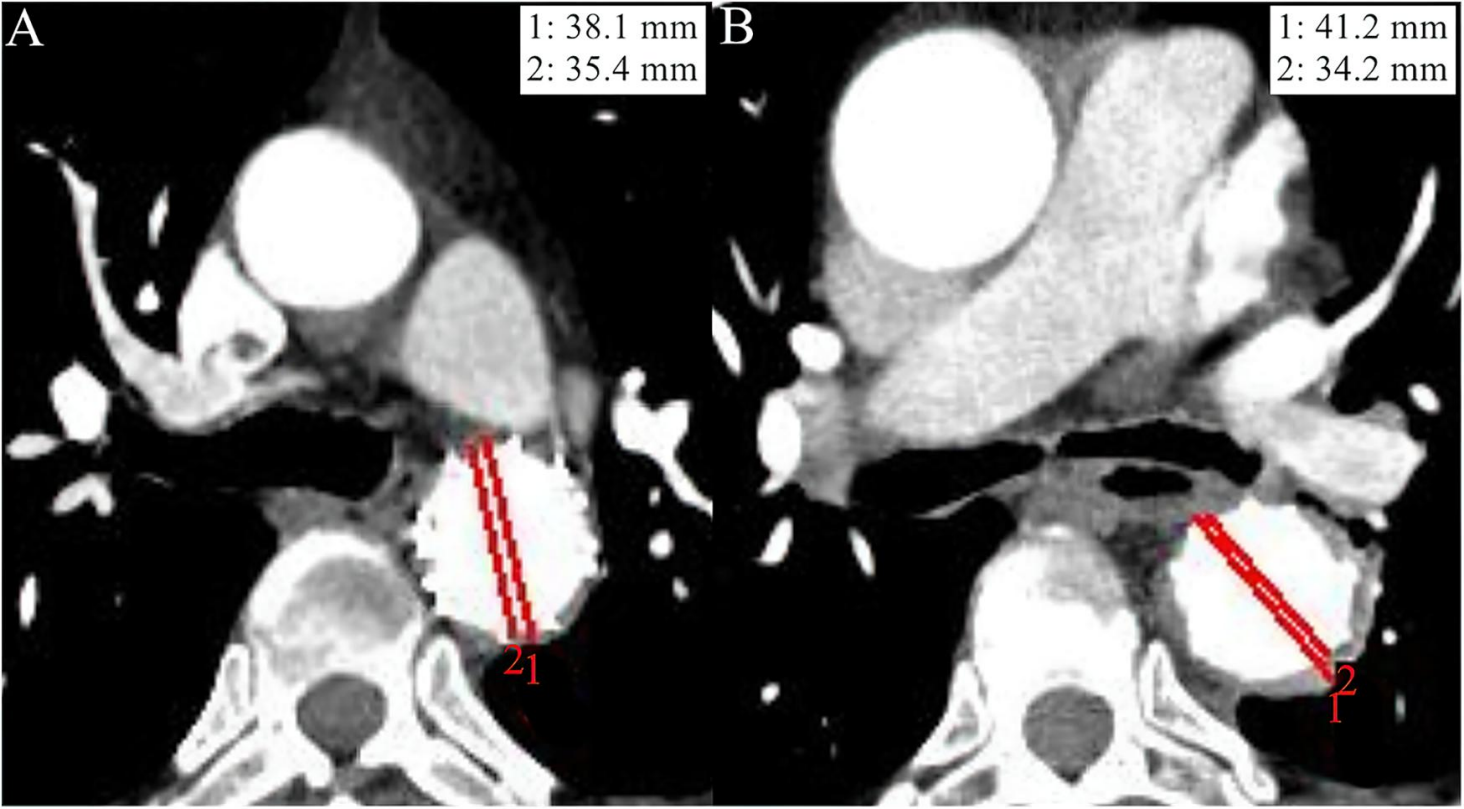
Imaging data from the aortic computed tomography angiography (CTA) of two study patients at the 1-year follow-up. The red line 1 indicates the total aortic diameter (TAD), while the red line 2 indicates the true lumen diameter (TLD). (A) An adult patient diagnosed with diabetes mellitus (DM) at admission had a TAD/TLD ratio of 1.08, indicating a high degree of aortic remodeling. (B) An adult patient without DM at admission showed relatively poor aortic remodeling degree as evaluated by a TAD/TLD ratio = 1.21, compared with patient A.
